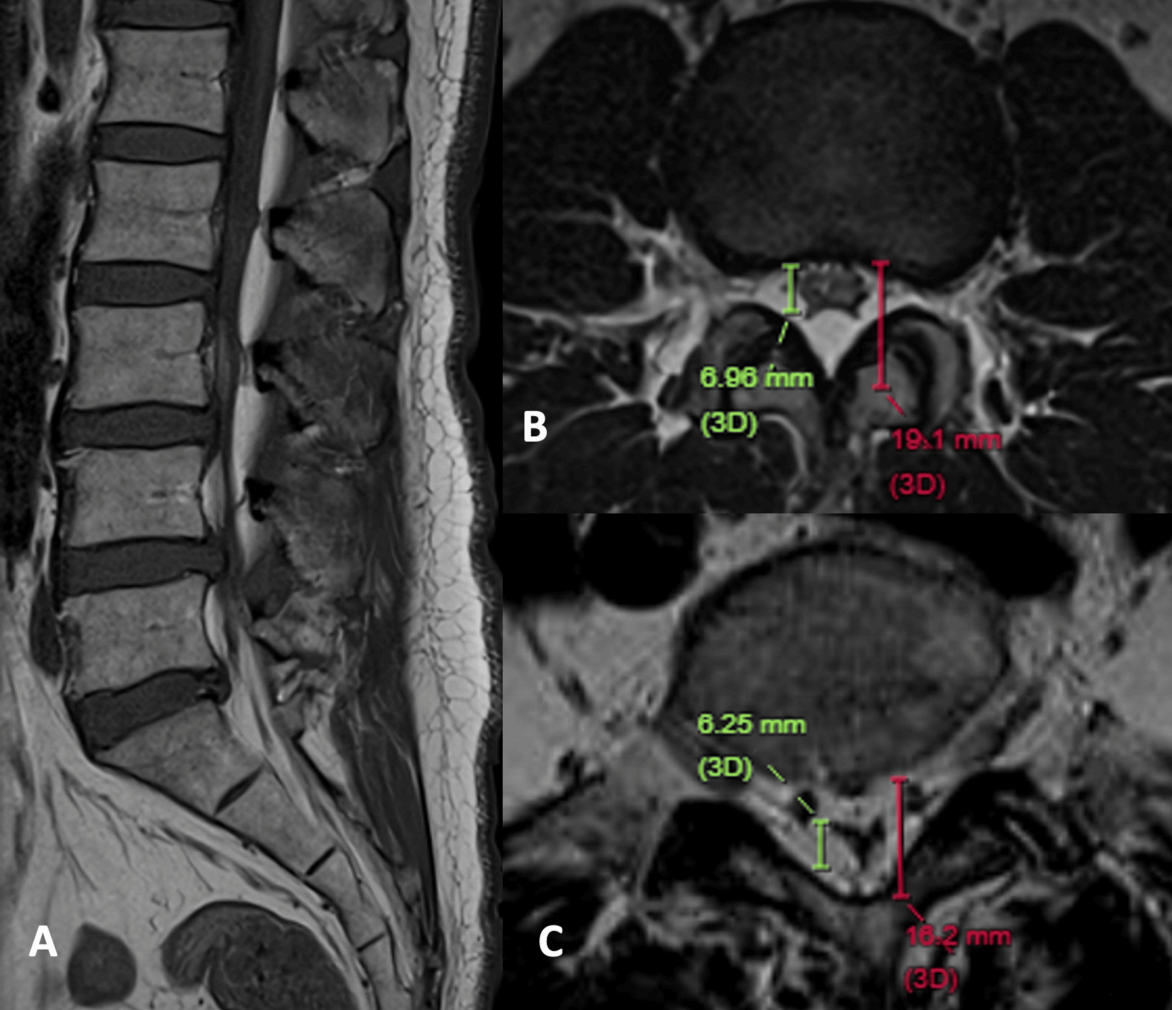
MRI of the lumbosacral spine of a 60-year-old man with chronic lower back pain and increasing spinal claudication. (A) Sagittal T1-weighted image shows a loss of lumbar lordosis and prominent epidural fat from L1 to S2 levels. (B) Axial T2-weighted image at L2/3 shows increased posterior epidural fat and degenerative changes, resulting in moderate spinal stenosis with polygonal dural sac (dura/fat ratio = 0.58, fat% = 63%). (C) Axial T2-weighted image at L5/S1 level revealed a Y-shaped dural sac from anterior and posterior epidural fat compression (dura/fat ratio= 0.63, fat% = 61%). Both levels are moderate SEL, with cauda equina crowding. MRI: magnetic resonance imaging; SEL: spinal epidural lipomatosis.
